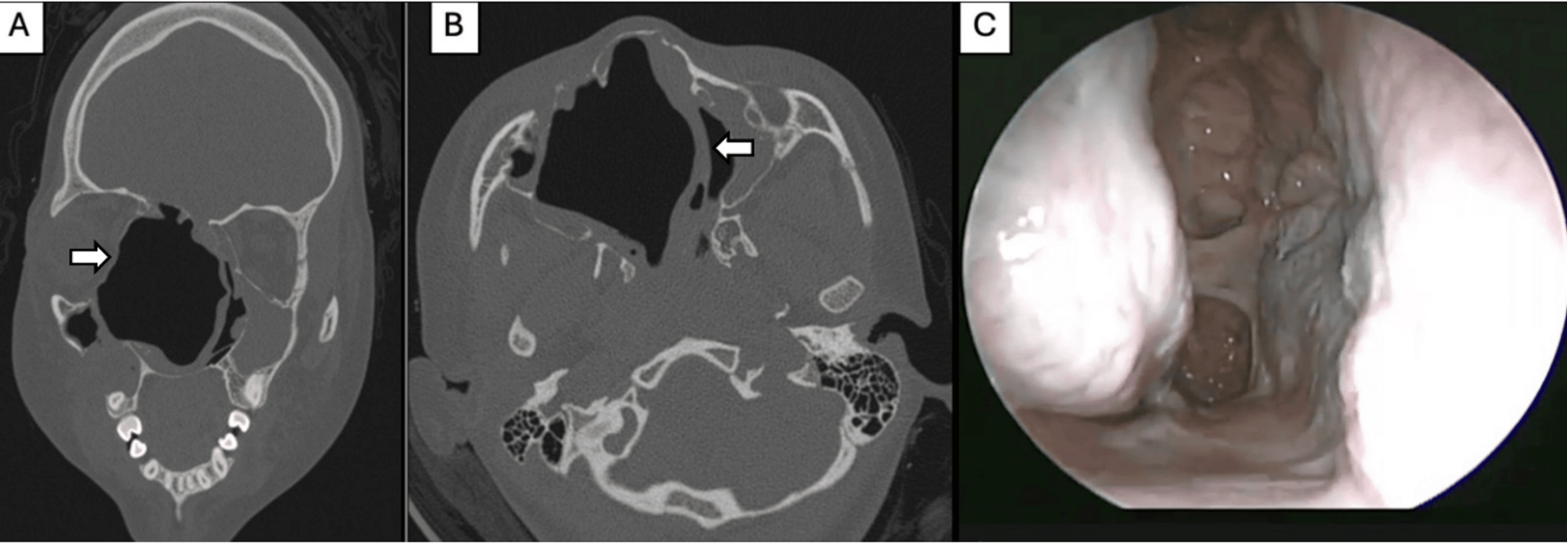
Case 2: Postoperative CT and nasal endoscopy of the right nasal cavity. A) Coronal view without tumor and orbital displacement to the right with lamina papyracea erosion (white arrow). B) Axial view of the nasal septum displaced to the left (white arrow). C) Nasal endoscopy of a patent right nasal cavity without signs of tumor recurrence up to the nasopharynx.
